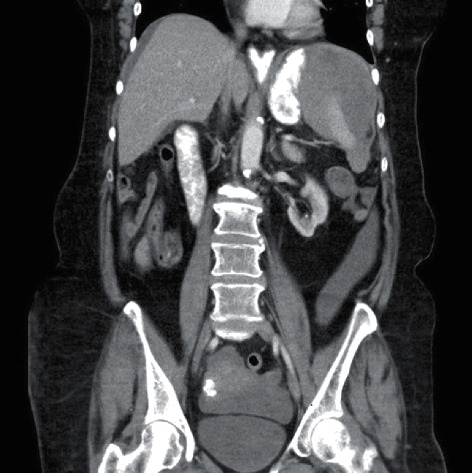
Coronal CT section of the abdomen and pelvis showing a large splenic hematoma.
